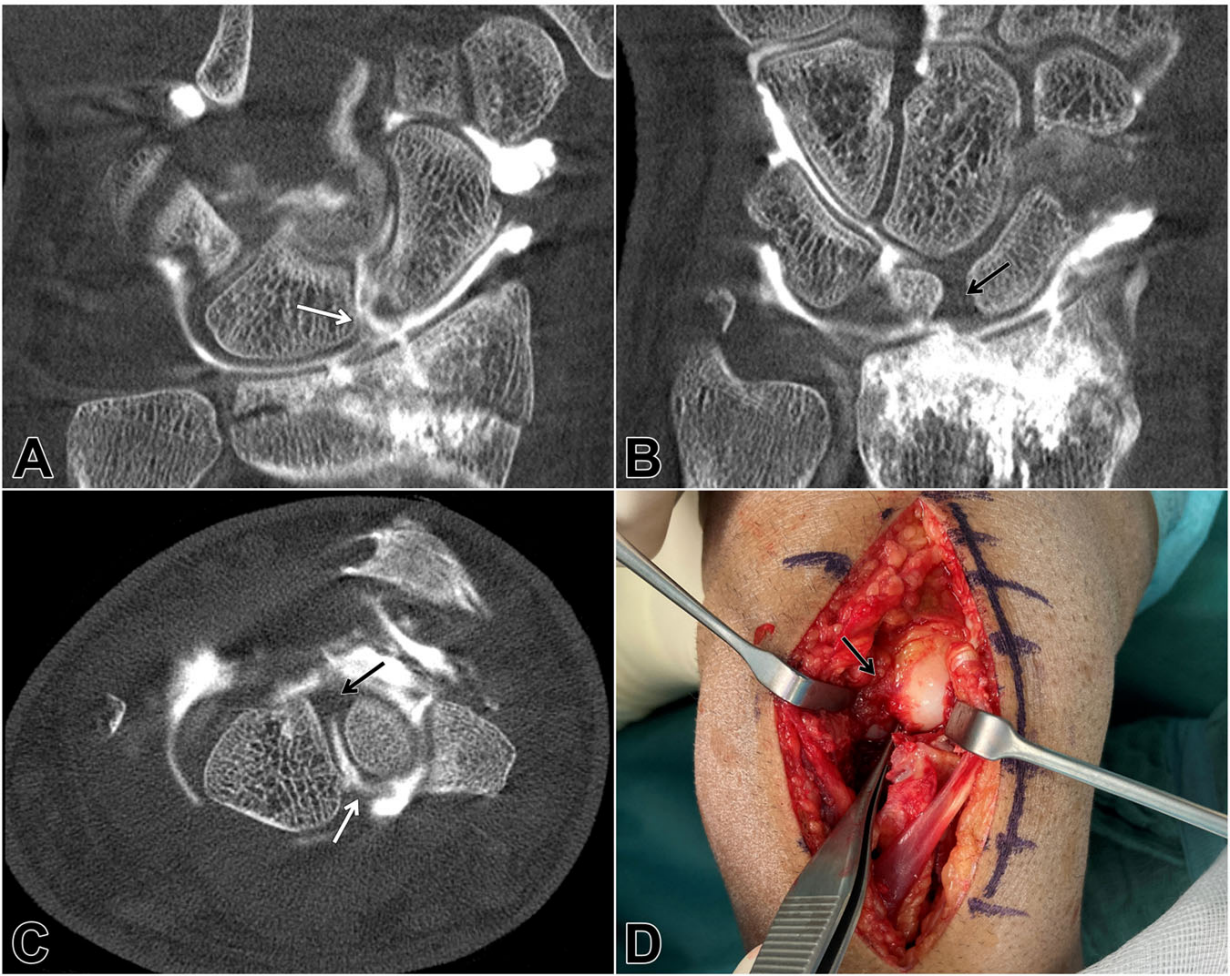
Cone-beam CT arthrography was requested for a 29-year-old man with an articular, multi-fragmentary radius fracture after a fall from a great height. CT images after articular contrast injection revealed a tear of the palmar scapholunate ligament (A: coronal, C: axial; *white arrows*), whereas the stabilizing dorsal segment was deemed intact (B: coronal, C: axial; *black arrows*). Undergoing open reduction and internal fixation of the radius fracture, no additional ligament suture or carpal transfixation was performed based on the integrity of the dorsal scapholunate ligament (D; *black arrow*)
